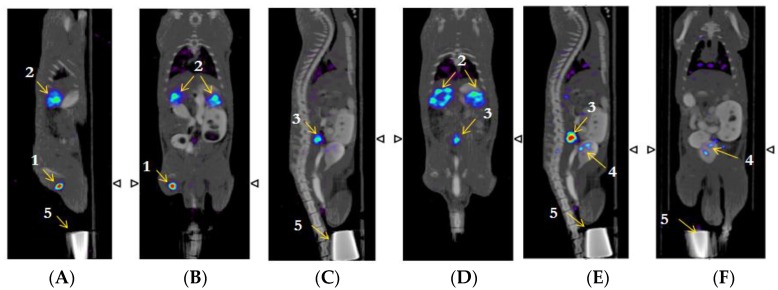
Biodistribution of β-NTx-DTPA-^67^Ga by molecular image (SPECT/CT) 3 h post-injection; image representative of four individual experiments. (A) and (B) are sagittal and coronal slices, respectively, showing uptake in the popliteal node (labeled as 1) and kidneys (labeled as 2); (C) is a sagittal slice demonstrating uptake in the lumbar node (labeled as 3); (D) is a different sagittal slice, showing accumulation in lumbar node and kidneys; (E) demonstrates accumulation in lumbar node and bladder (labeld as 4); (F) shows the accumulation in bladder. Number 5 shows the injection site, masked by a lead shield.
